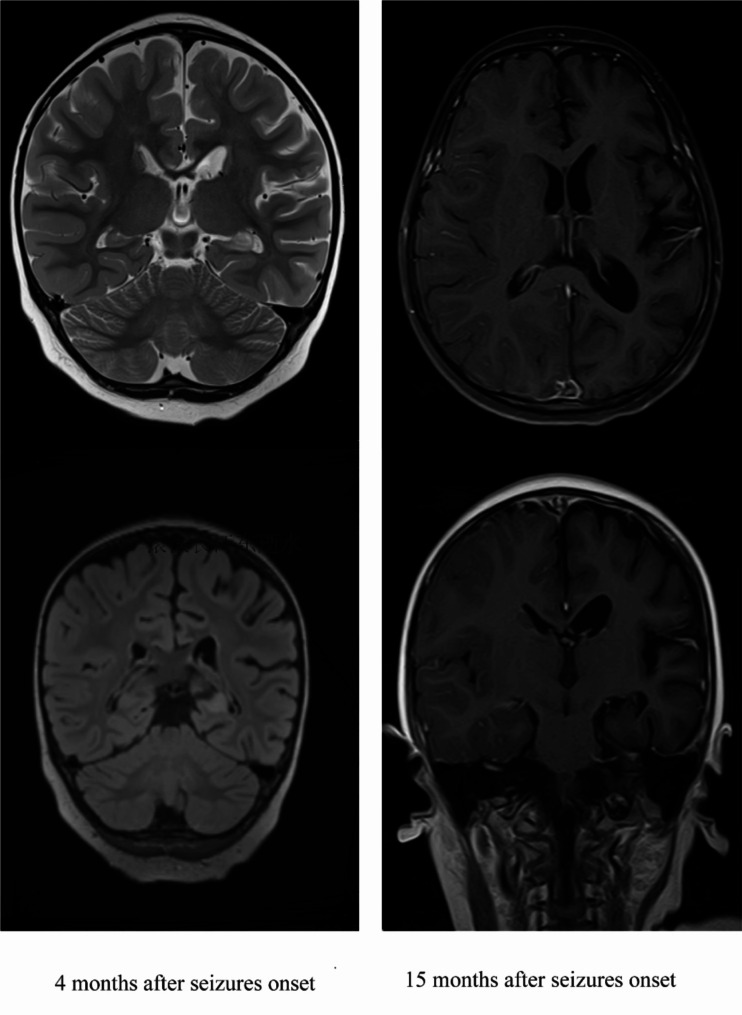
Brain MRI of case 1. MRI at 4 months after seizures onset showed smaller gyrus of the left frontal temporal parietal occipital lobe, with widening of cerebral fissure, slightly larger left ventricular temporal angle, smaller size and slightly T2/FLAIR hyperintensity signals of the left hippocampus. MRI at fifteen months after seizures onset showed atrophy of the left cerebral hemisphere and reduced lesion of the T2/FLAIR hyperintensity of the left hippocampus
